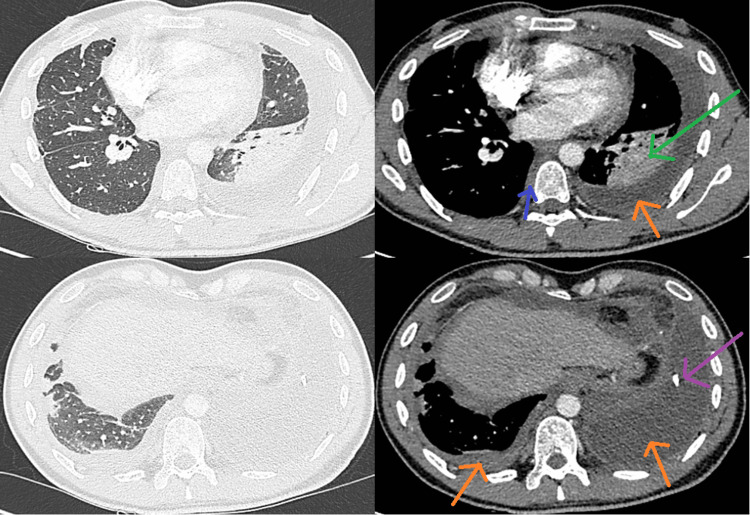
Chest computed tomography after evacuation of the pleural effusion showed bilateral small pleural effusions (orange arrows) with a right pleural thickening (blue arrow) and partial trapping of the left lower lobe (green arrow). A pleuro-catheter is noticed inside the left pleural effusion (purple arrow).
